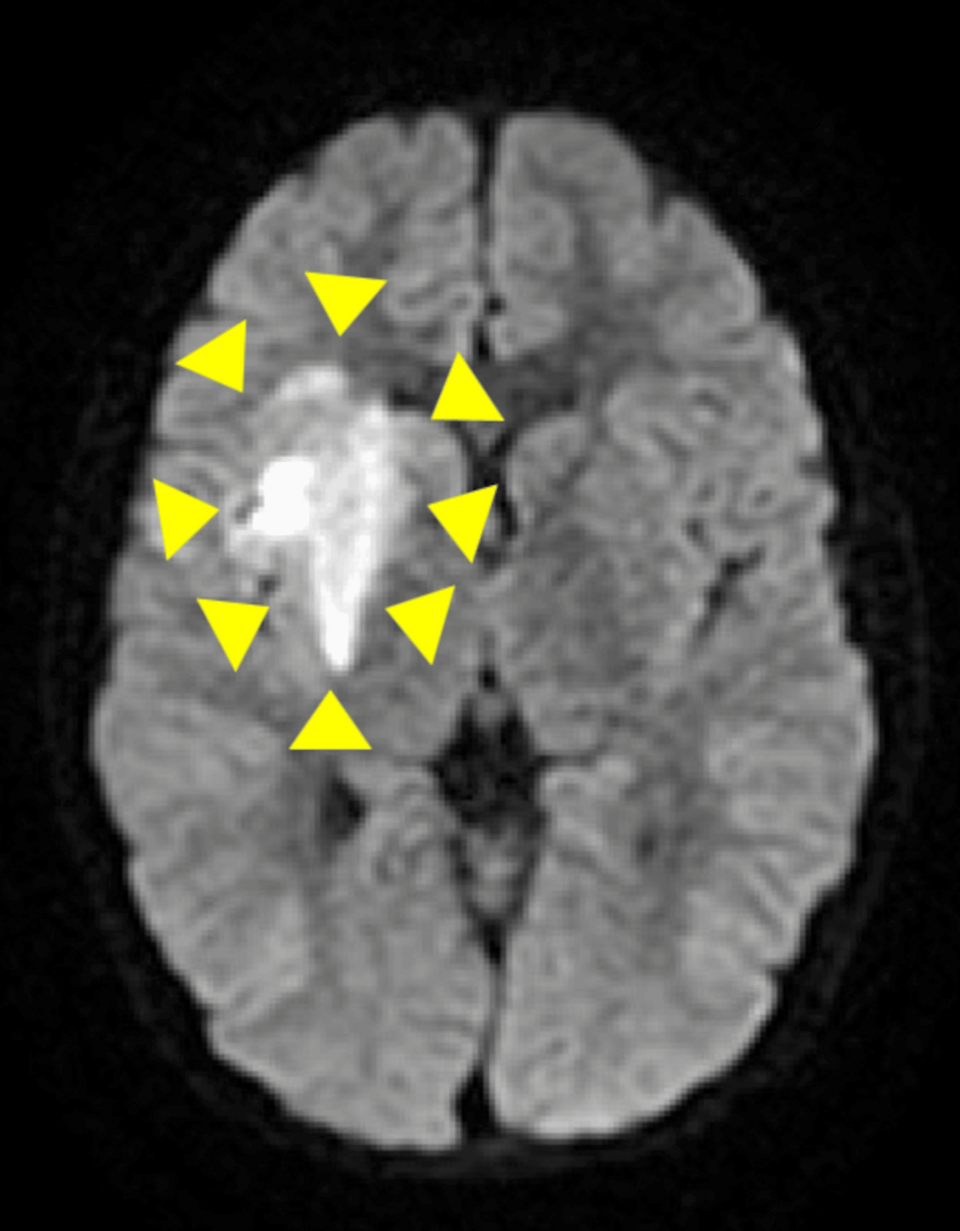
Diffusion-weighted MRI image of the head on day 2 of admission. There is an area of high signal, indicative of cerebral infarction in the right middle cerebral artery (area surrounded by yellow arrowheads).
